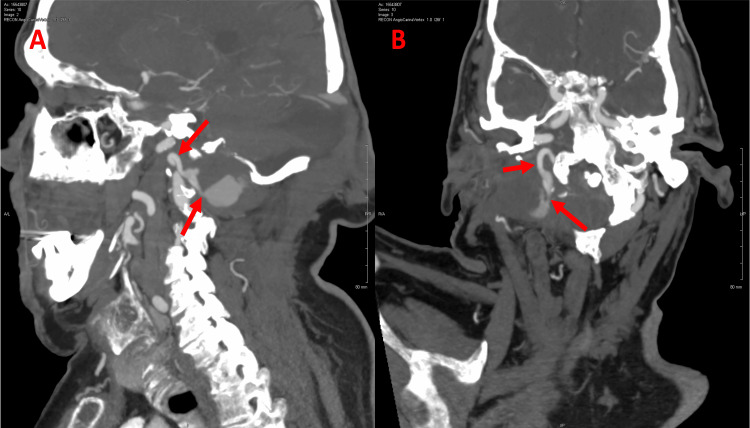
Computed tomography angiography of the patient's head and neck arteries - pre-treatment. (A) Sagittal section; (B) axial section. Communication between the right vertebral artery and the mass with contrast material leaking from the vessel to the lesion (red arrows).
